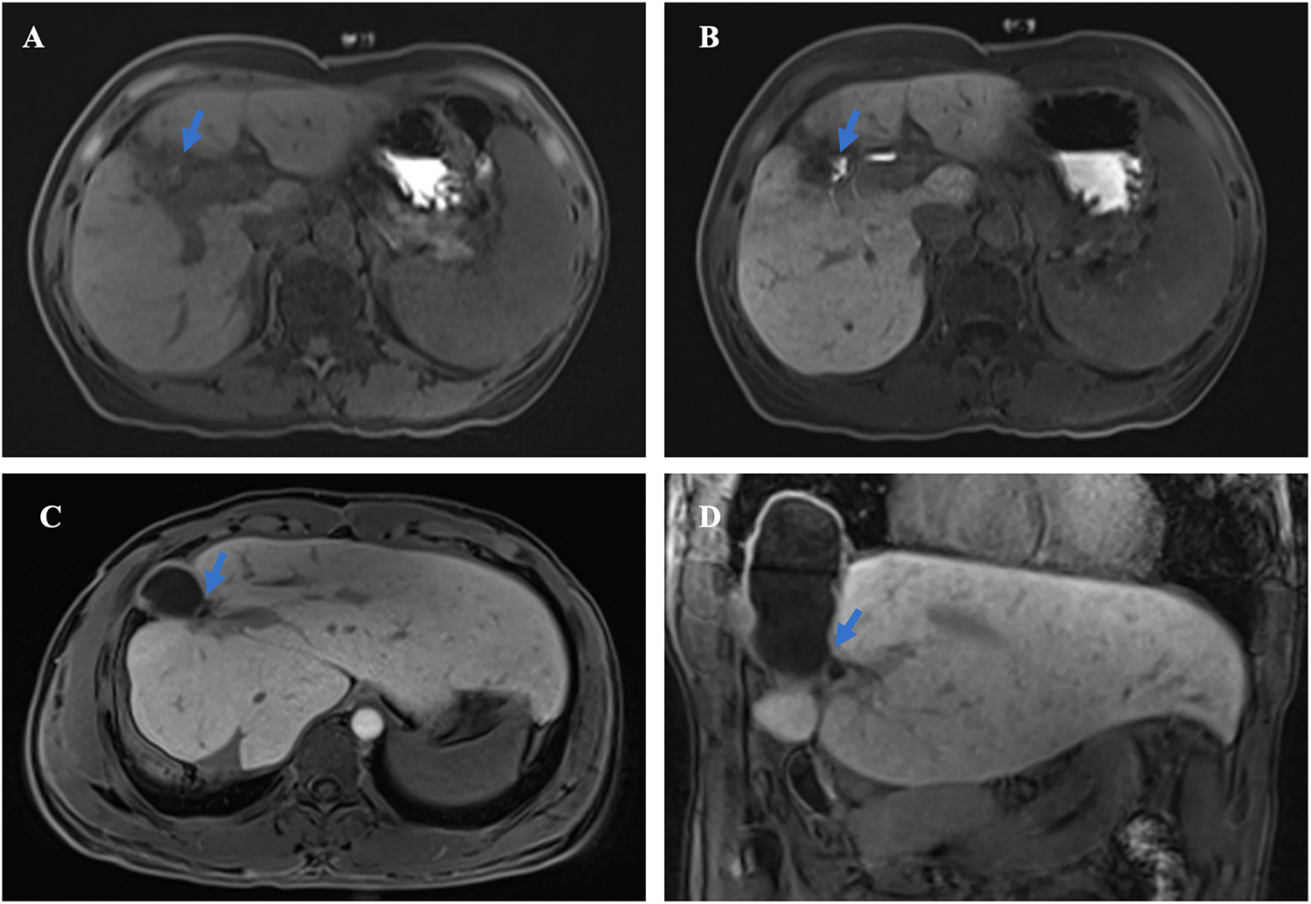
Bile leakage and bile lake. A A 49-year-old female patient with bile leakage after partial hepatectomy. Axial pre-contrast phase of MRI shows no significant hyperintensity. B The same patient in A. Axial hepatobiliary phase of MRI shows the active bile leakage with hyperintensity. C A 28-year-old male patient with continuous cutaneous bile leakage after right hemi-hepatectomy. The axial hepatobiliary phase of MRI shows the bile lake near the left bile duct with no contrast filling in and the connection between the cyst and the left bile duct (arrow). D The same patient in C. The coronal hepatobiliary phase of MRI shows the connection between the cyst and the left bile duct (arrow)
